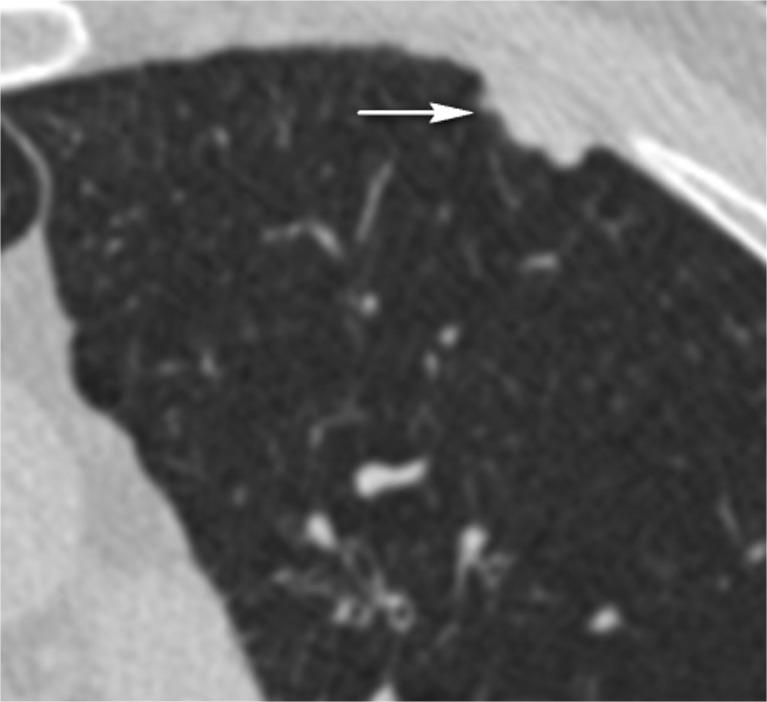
Example of a left upper lobe lesion (white arrow) identified on CT by a radiographer, but dismissed by the concurrent reading radiologist as a false-positive finding. The patient also had other calcified pleural plaques (not shown) consistent with previous asbestos exposure
